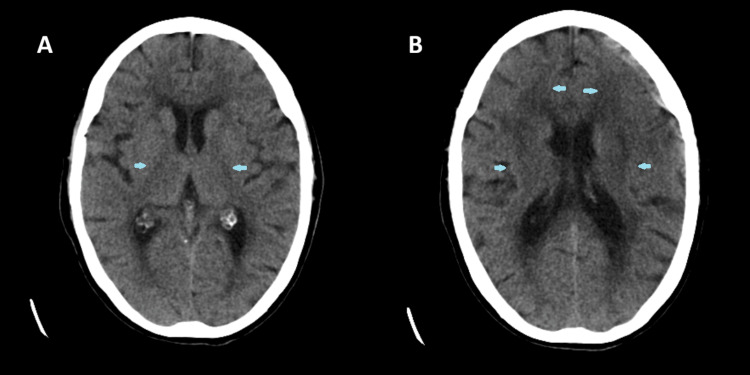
CT head for the patient Non-contrast axial CT images of the brain show no evidence of acute intracranial hemorrhage or large territorial infarction. The scans (A) and (B) demonstrate mild diffuse cerebral atrophy and chronic small-vessel ischemic changes (marked in blue arrows).
